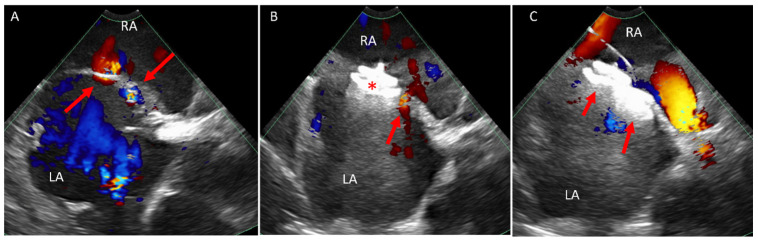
(A) Color Doppler with the ICE catheter in the right atrium showing evidence of two atrial septal defects (red arrows). (B) Color Doppler image post deployment of the first atrial septal defect device occluder (asterisk) with residual left to right shunt (red arrow). (C) Color Doppler showing two ASD occluder devices deployed (red arrows) with no residual shunt. ASD, atrial septal defect; ICE, intracardiac echo; LA, left atrium; RA, right atrium.
